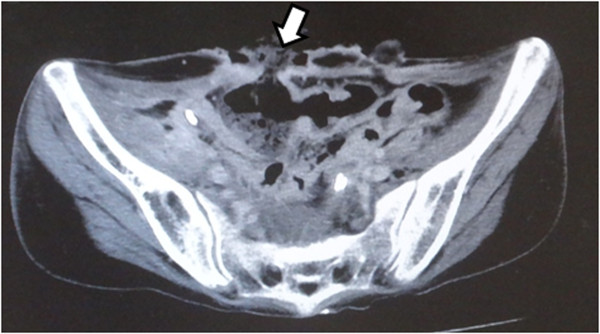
Computed tomography scan showing the necrotic colon (arrow).
